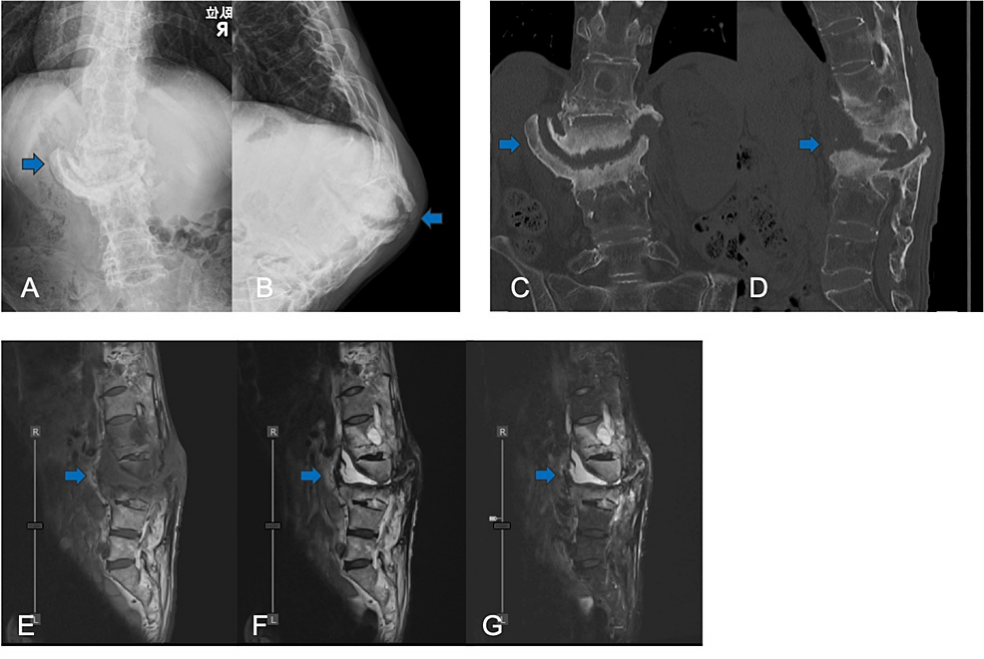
(A, B) Radiograph in the sitting position showing scoliosis, kyphosis, and spinal instability (blue arrows). (C, D) CT showing destructive change and large osteophyte on L2/3 (blue arrows). MRI sagittal T1-weighted (E), T2-weighted (F), and STIR (G) images showing continuous fluid from L2/3 to the posterior fistula (blue arrows).
